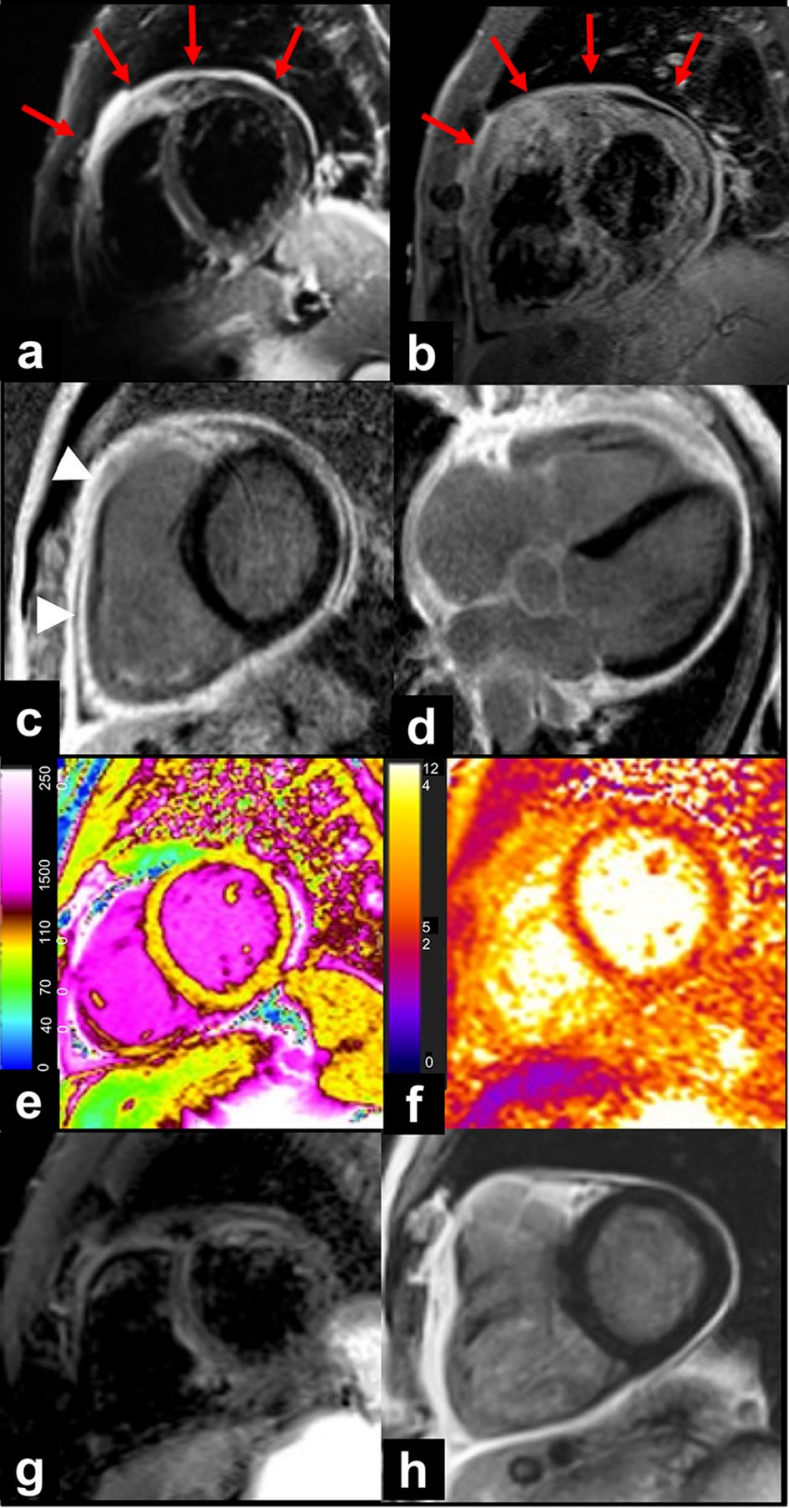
Vaccine-associated pericarditis. 57-year-old male admitted to ED for chest pain, fatigue and dyspnea after 8 days following second dose of Comirnaty vaccine. The first CMR (a–f), performed for the clinical suspicion of myocarditis, showed edematous thickening and enhancement of pericardial layers (red arrows) respectively on STIR T2-weighted (a) and fat-suppressed turbo spin echo T1-weighted (b) images. Absence of myocardial injury and pericardial enhancement (arrowheads) has been detected on late gadolinium enhanced images acquired on short axis (c) and horizontal long axis (d) view. Myocardial native T1 (e) and T2 (f) values were within normal range on corresponding maps. At 13-weeks follow-up CMR, there were neither pericardial fluid nor edema on STIR images (g) nor myocardial LGE areas (h)
